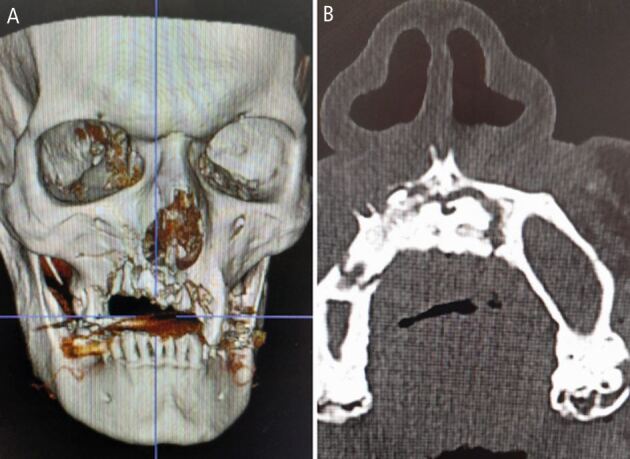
Case 1. (A) CT imaging 3D reconstruction. (B) CT axial view showing extensive maxillary osteonecrosis with a moth-eaten appearance, significant resorption of the alveolar process resulting in loss of most anterior teeth, extension into the palatine process, involvement of both maxillary sinus walls and multiple cortical defects with sequestered bone foci
